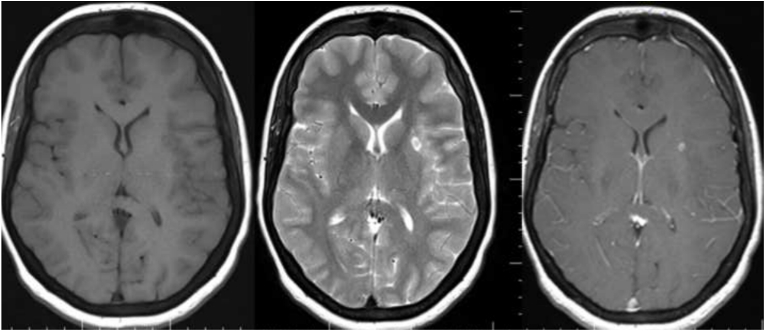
Noncaseating granuloma. (A) Tuberculoma in the left basal ganglia is hypointense in the T1-weighted axial image, (B) hyperintense in the T2-weighted axial image. (C) Nodular contrast enhancement of the tuberculoma on the post-contrast image.
